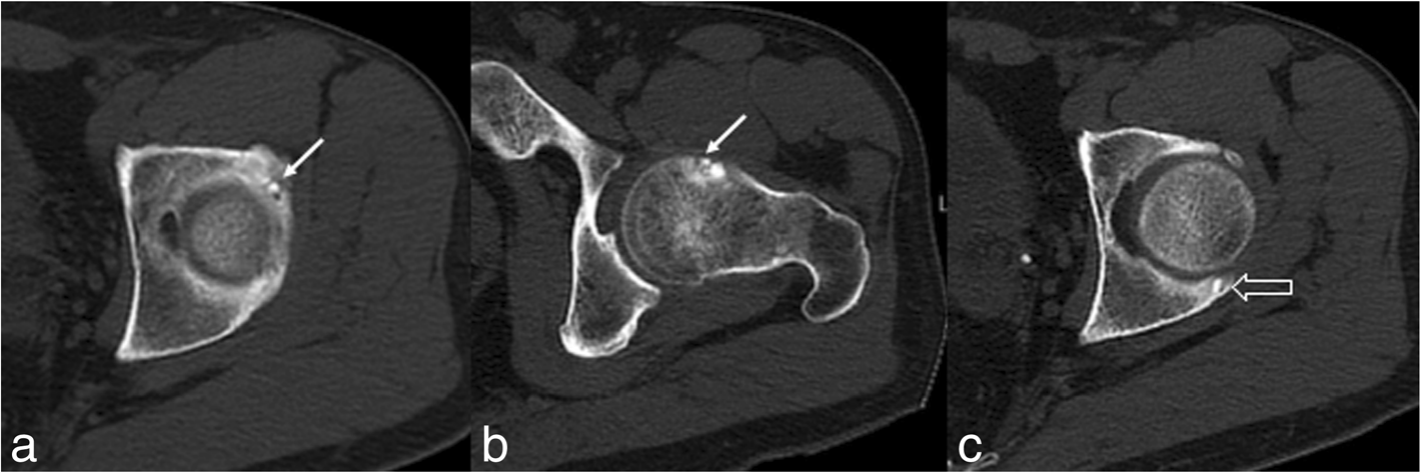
After two-years history of left hip pain, the patient underwent pelvis CT scan revealing the synchronous presence of two intracortical bone lesions consisting of a radiolucent nidus with a central punctiform hyperdensity and sclerosis of the adjacent bone, located in the left anterior acetabulum (a, arrow) and femoral neck (b, arrow), suspicious for OO. A third smaller lesion of the posterior acetabulum, with the same imaging findings (c, void arrow) was initially overlooked
